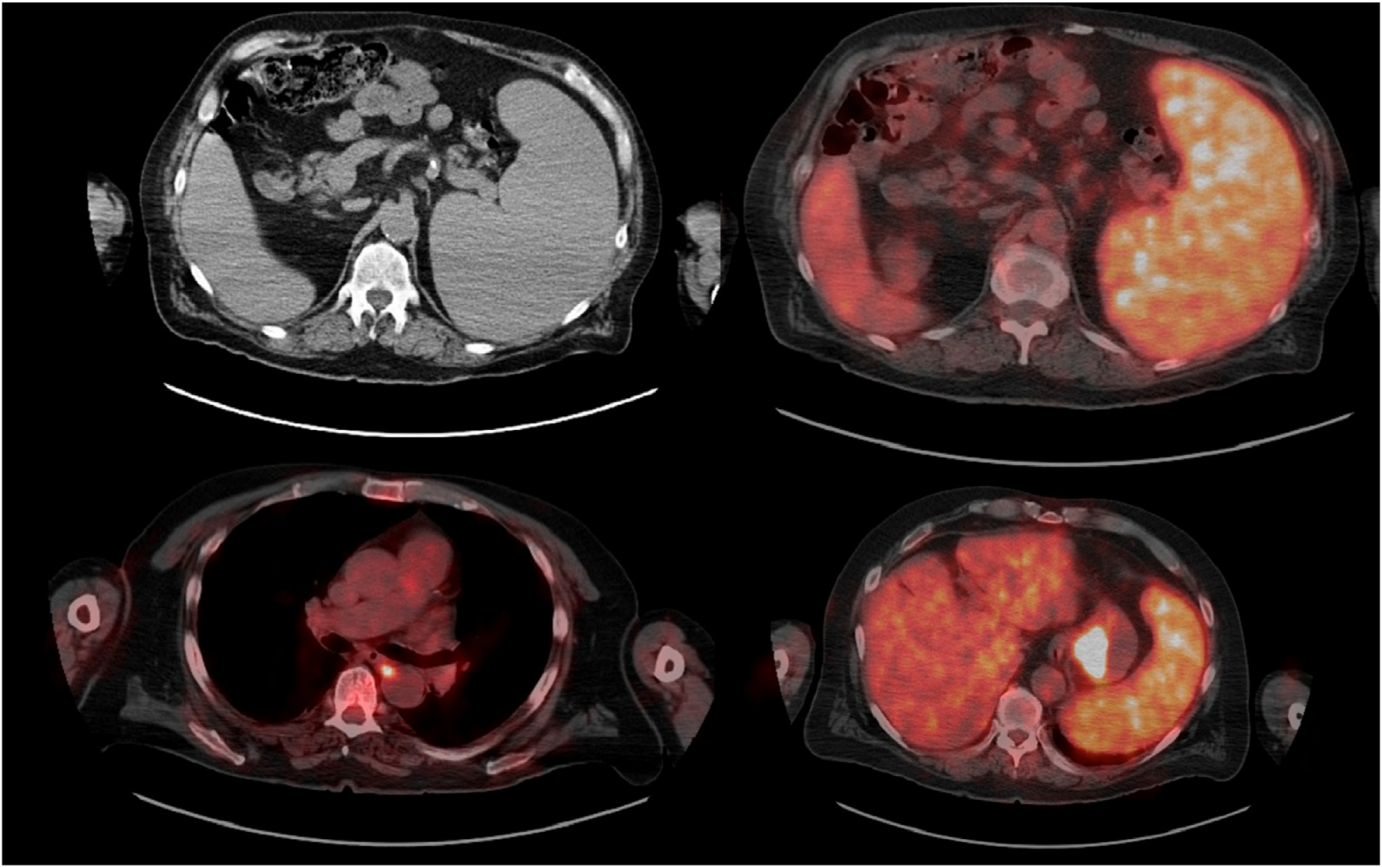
Massive hepatosplenomegaly along with hypermetabolic foci seen on positron emission tomography/computed tomography scan in the proximal stomach (standardized uptake value [SUV], 14.0) and between the esophagus and descending aorta (SUV 4.9).
